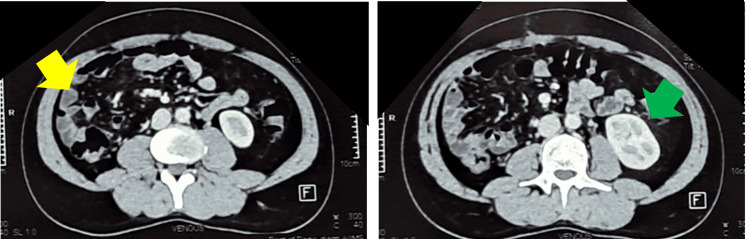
Postoperative contrast-enhanced computed tomography (CECT) showing no residual or recurrent disease. Yellow arrow: postoperative bed; green arrow: normal opposite kidney.
